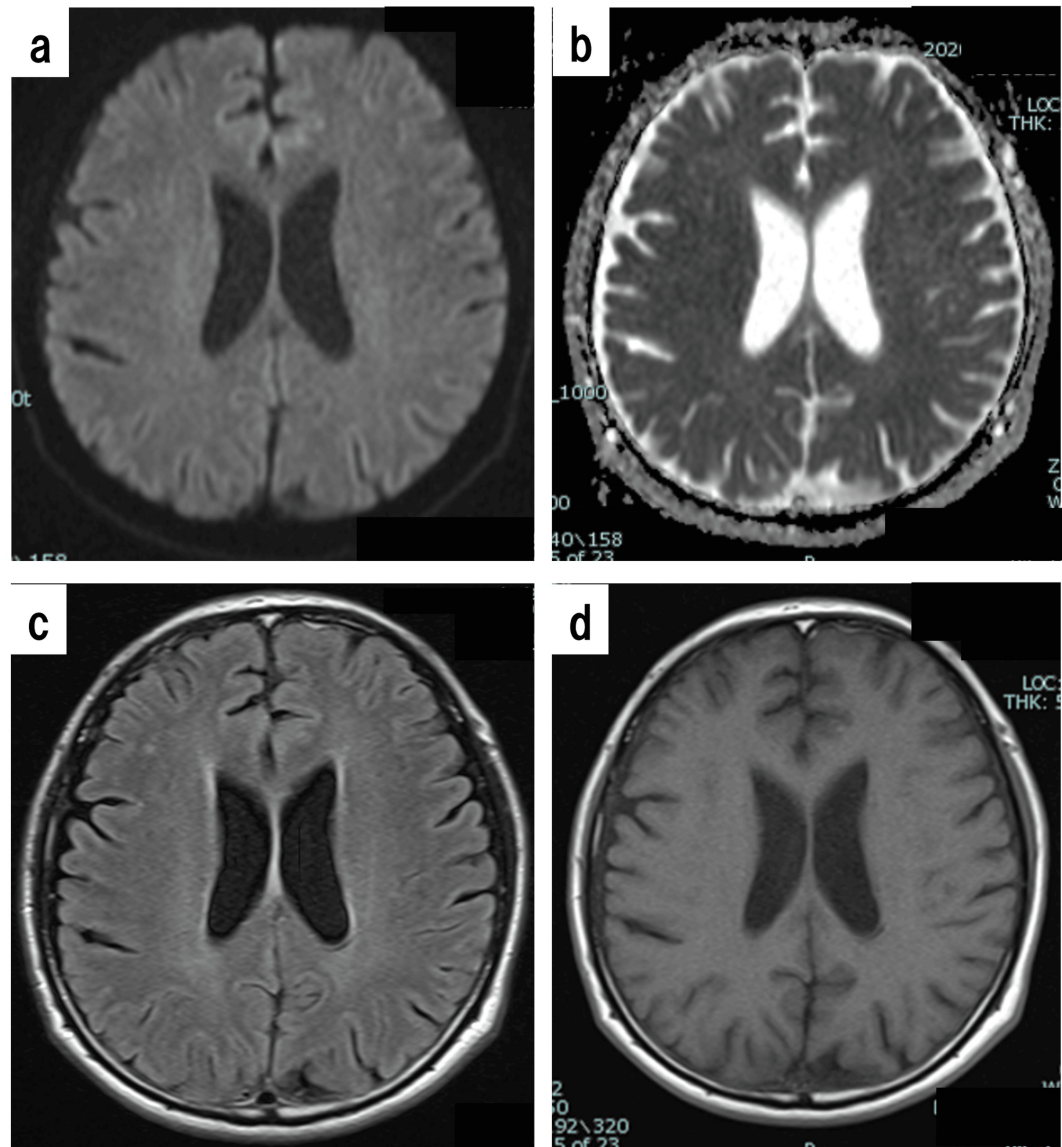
Brain MRI performed on the 6th day after discharge. a: Axial diffusion-weighted image; b: Apparent diffusion coefficient (ADC) image; c: Fluid-attenuated inversion recovery (FLAIR) image; d: T1-weighted (T1WI) image. No abnormalities were found on the brain MRI scans.
